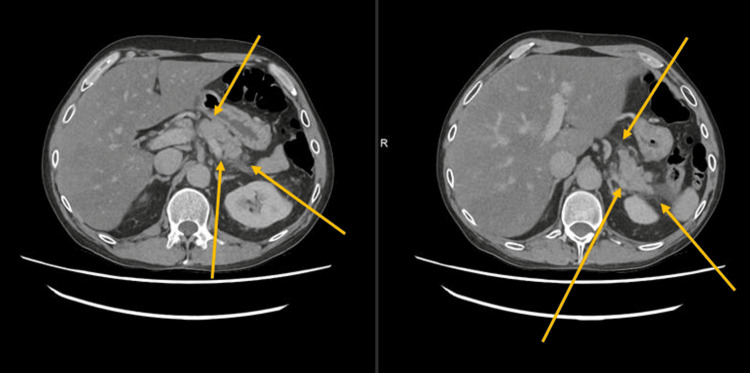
CT scan of the abdomen of Case 2 revealed features suggestive of acute pancreatitis without pancreatic necrosis. The arrows highlight radiological features consistent with acute pancreatitis, including pancreatic enlargement and surrounding peripancreatic inflammatory changes.
